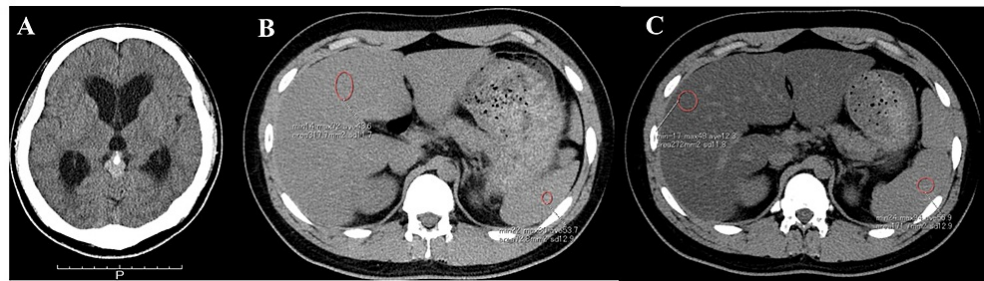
Brain and abdominal CT. 1A. Brain CT: Brain tumor with calcification in the pineal region, with narrowing of the midbrain aqueduct and third and lateral ventricle enlargement. 1B. Initial abdominal CT: CT revealed a slightly lower attenuation of the liver/spleen (L/S) ratio of 0.81. 1C. Abdominal CT at the time of consultation: CT showed that the L/S ratio was extremely decreased to 0.21, 41 days later, following the period of inactivity.
